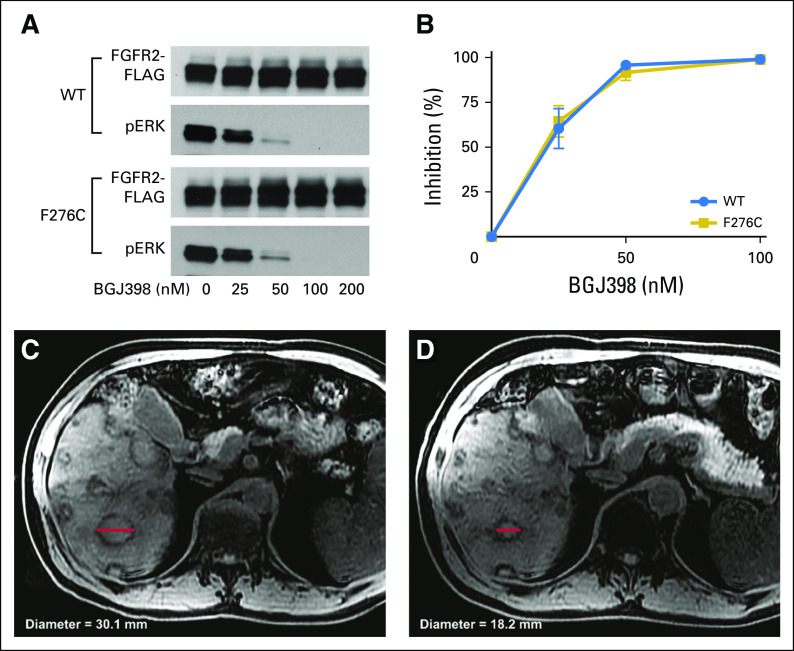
Response of F276C FGFR2 to BGJ398 treatment. (A) KMCH-1 cells were transfected with F276C and wild type (WT) by using a 3.5/5 DNA ratio, respectively, to normalize expression levels. After 1 day, cells were incubated with serum-free medium with 20 ng FGF2 for 16 hours. Cells were then treated with BGJ398 (0 to 200 nM) or vehicle (dimethyl sulfoxide) at 37°C for 3 hours. Cell lysates were then analyzed by Western blot for FGFR2-FLAG and phospho-ERK (pERK). (B) Quantitation of dose response to BGJ398 for experiments as shown in (A); n = 3 for each BGJ398 concentration. Values are mean ± SE and expressed as percent inhibition of pERK signal compared with cells with no BGJ398. Response of FGFR2 F276C–containing tumor to BGJ398 in (C) September 2015 (pretreatment, with magnetic resonance imaging showing a 30.1-mm tumor diameter [red line]) and (D) October 2015 (postinitiation of treatment, with pan-FGFR inhibitor BGJ398 magnetic resonance imaging showing tumor shrinkage to an 18.2-mm diameter [red line]).
